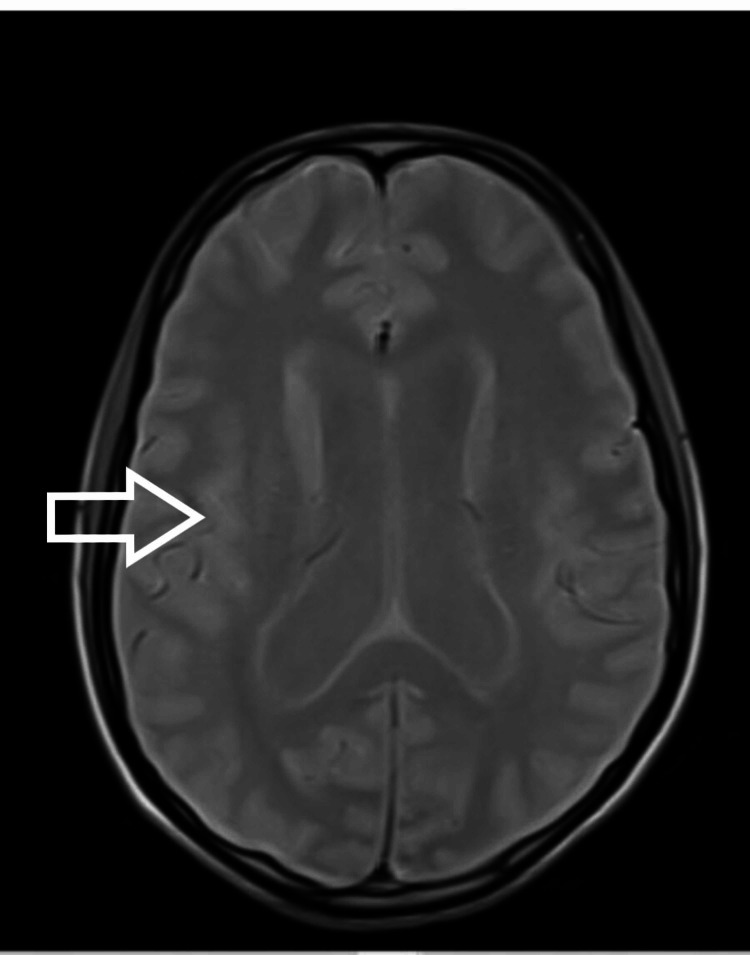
MRI brain showing evidence of hydrocephalus (A, B) with leptomeningeal enhancement on the post-contrast image (C) - view 3 MRI: magnetic resonance imaging
